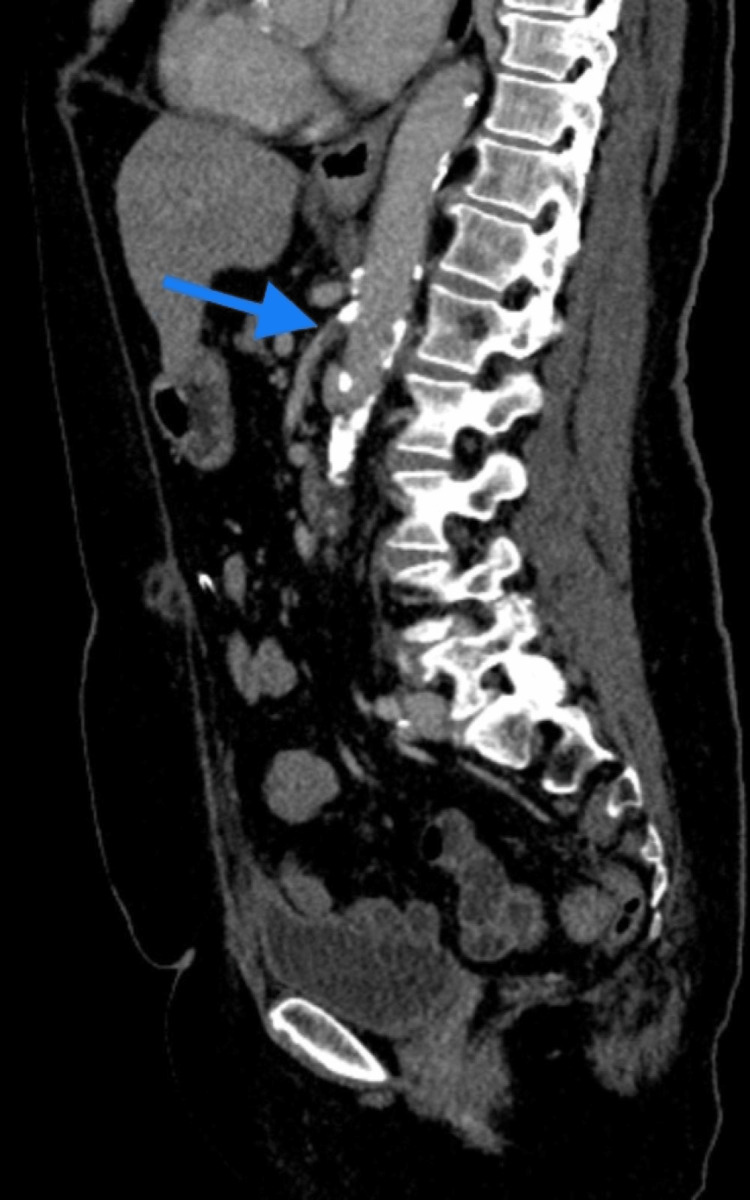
CT angiogram of the abdomen (sagittal reconstruction) demonstrates complete occlusion of the proximal superior mesenteric artery (blue arrow).
